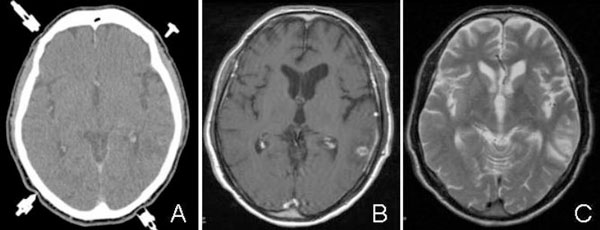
(A) Contrast-enhanced axial CT scan showing a round lesion in the left temporal lobe. (B) Contrast-enhanced axial T1-weighted MR image shows a sharply defined ring enhancement. (C) Axial T2-weighted MR image demonstrates a perifocal edema.
